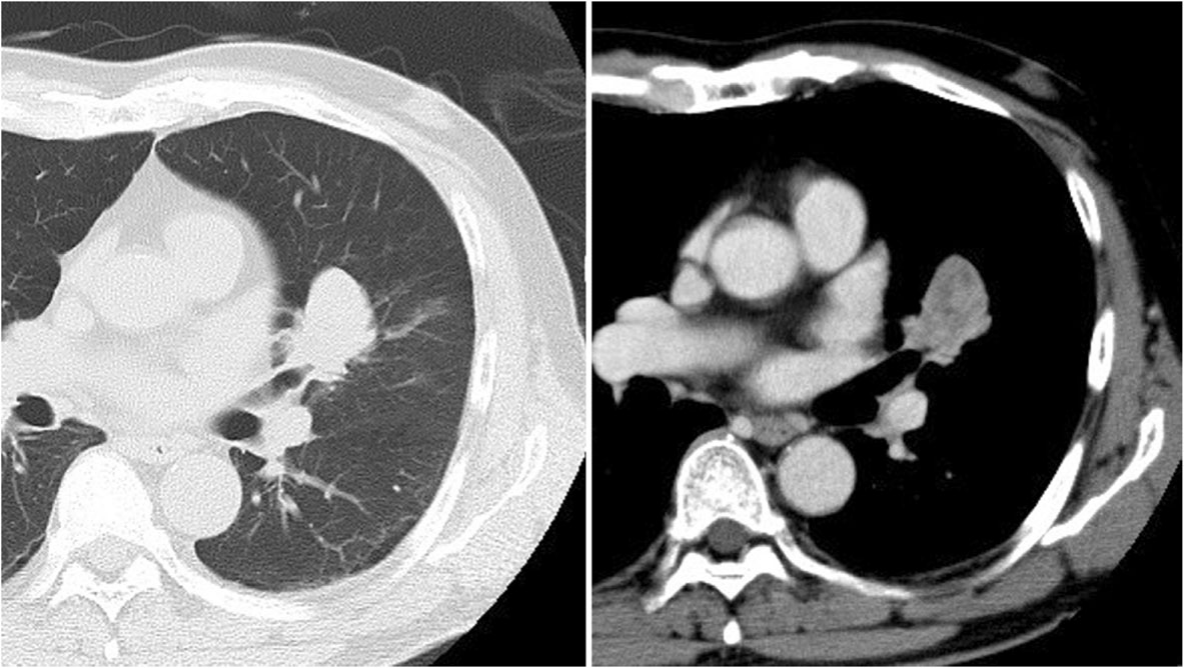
A 3.6 × 2.8 cm mass with a clear boundary and heterogeneous contrast enhancement was present in the left upper lobe on chest CT
